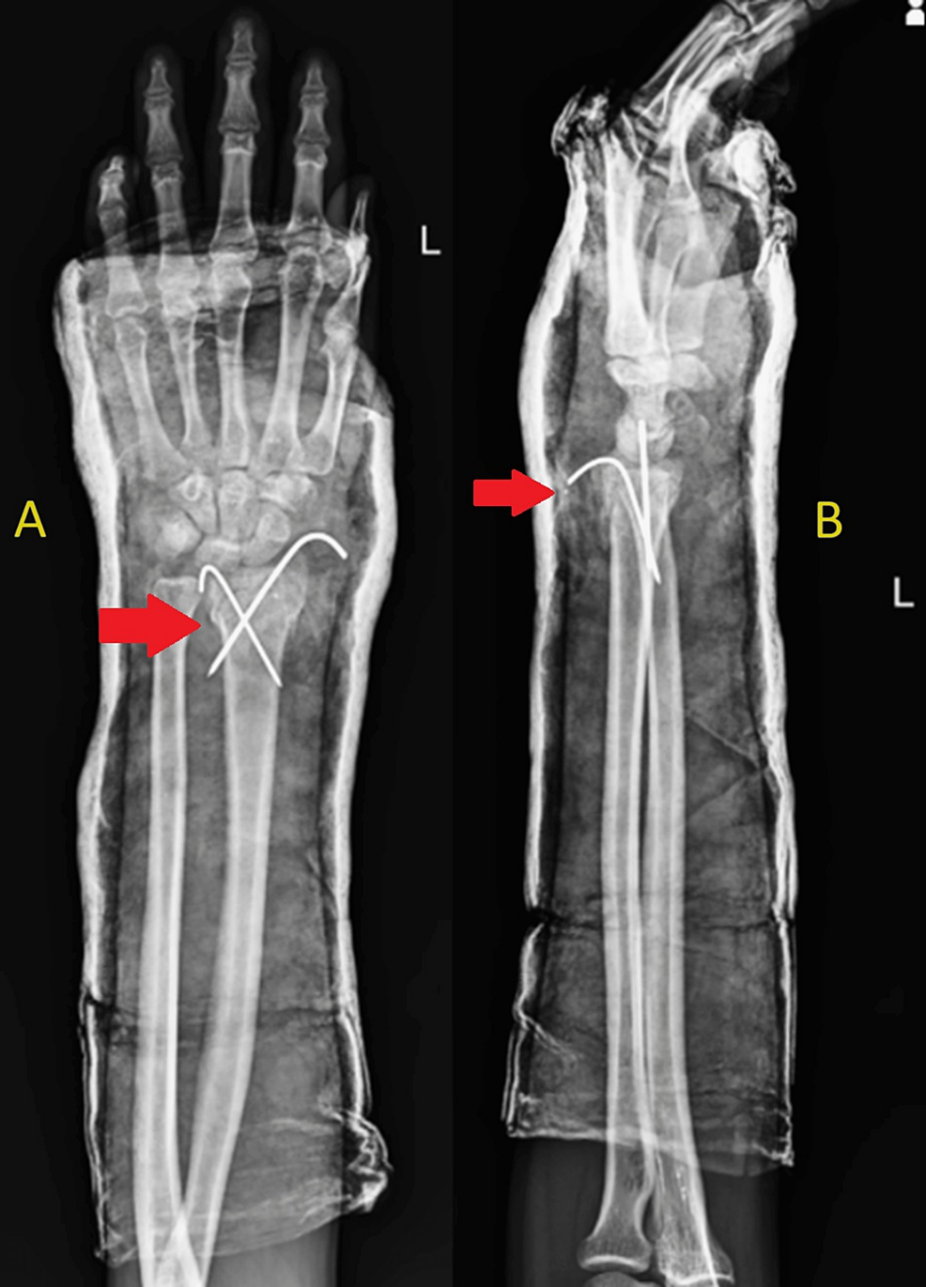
Immediate postoperative X-ray of the left wrist in anteroposterior (A) and lateral (B) views showing distal end of radius fracture with two K-wires in situ with overlying cast application. No signs of synovitis and juxta-articular osteoporosis.
